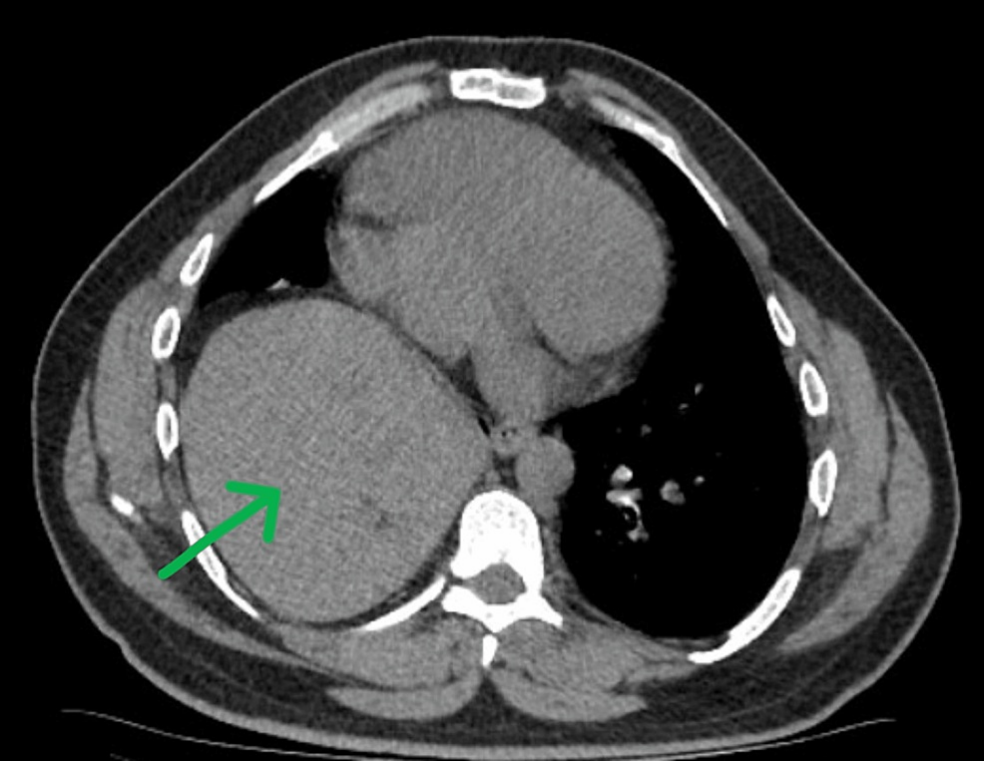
Axial section of the CT scan of the chest showing herniation of the liver into the thorax The liver (green arrow) can be seen next to the dextrocardic heart.
